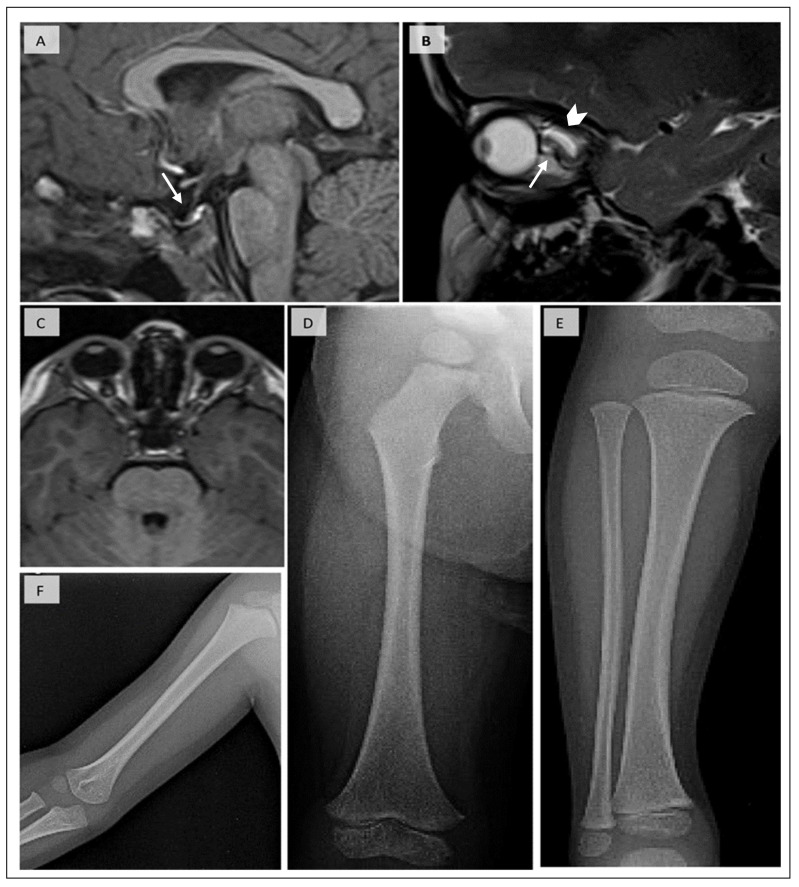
(A) T1-w midsagittal image shows hypoplasia of the anterior pituitary with a concave surface and almost empty sella appearance (arrow). The stalk is normal, and the posterior pituitary is orthotopic. (B) T2-w sagittal image demonstrates tortuosity of the optic nerve (arrow) and dilatation of the subarachnoid space surrounding its anterior portion (arrowhead). (C) T1-w axial image depicts bilateral symmetrical microphthalmia. X-rays of the (D) femur, (E) tibia, and (F) humerus show increased cortical thickness of the diaphysis, with mild narrowing of the medullary cavities. Relative flaring of the proximal tibial metaphysis.
